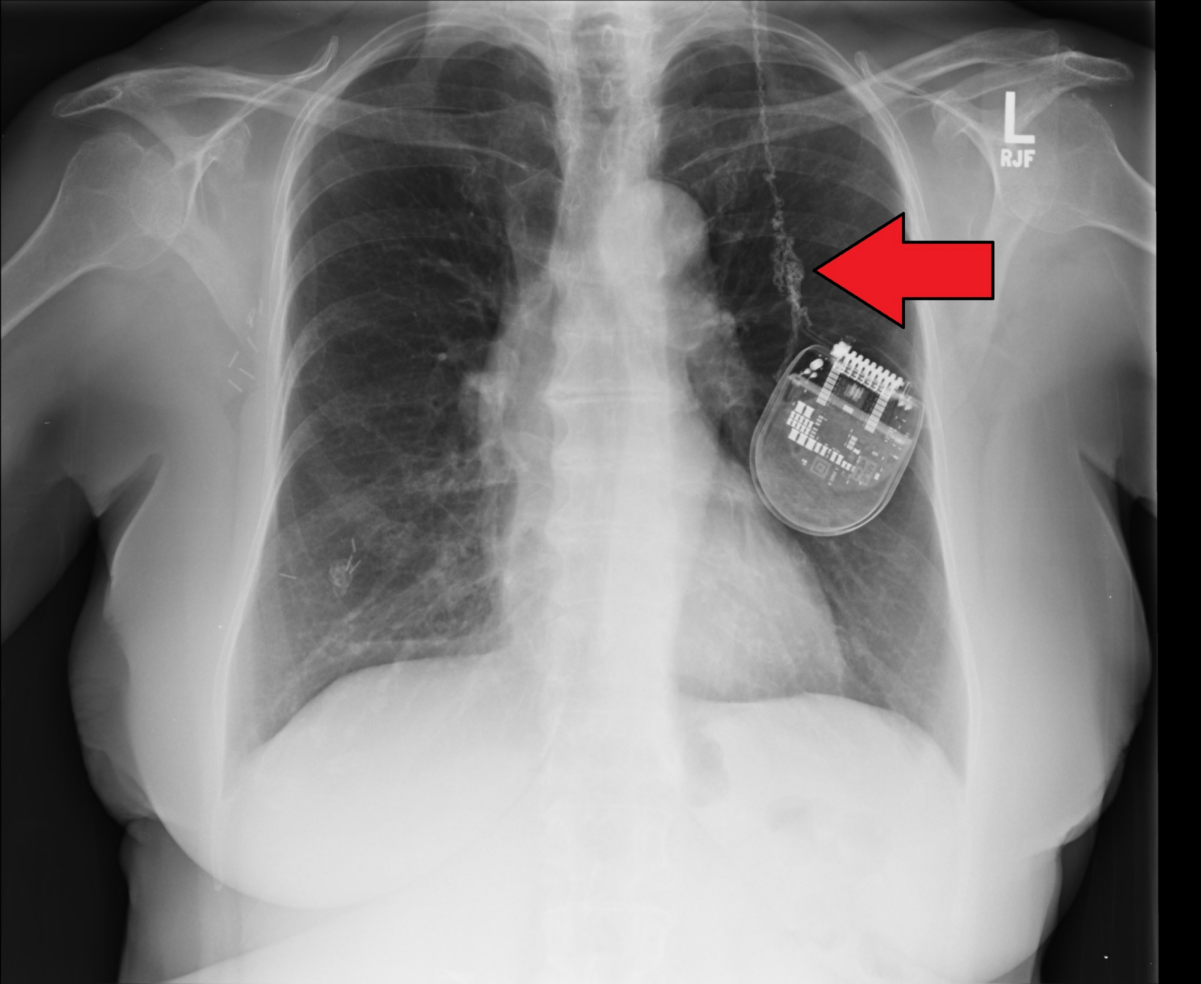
Chest X-ray demonstrating coiled leads on the left
